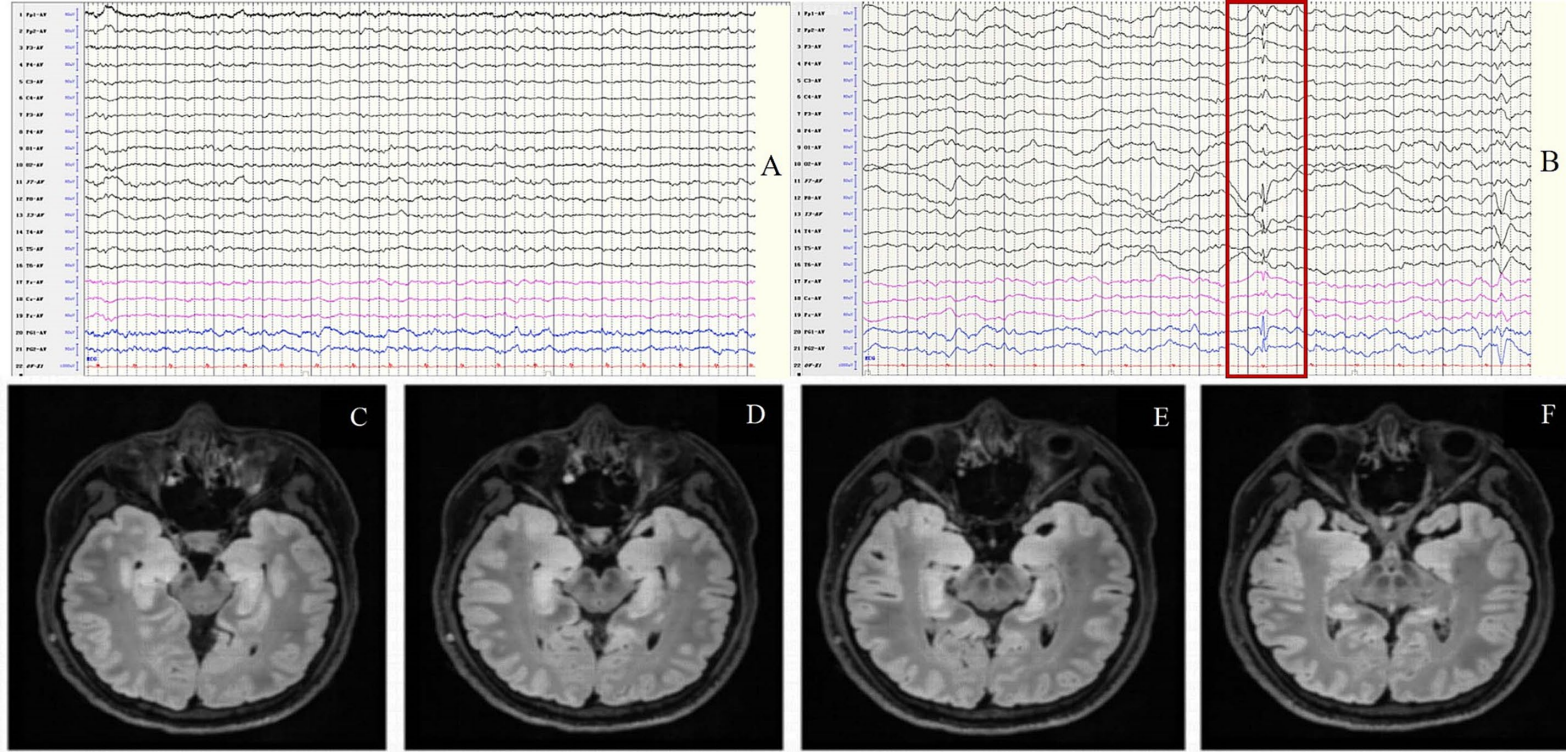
Case 51, female, 33 years old, who was diagnosed with anti-GAD65 encephalitis. (A,B) Fifteen days after onset and before immunotherapy, EEG showed focal slow waves in the background activity (A) and spikes in bilateral anterior temporal regions (B); (C–F) Thirty days after onset and before immunotherapy, brain MRI showed swelling and T2 FLAIR hyperintensity in the bilateral hippocampus. The red border signifies the spikes in bilateral anterior temporal regions.
